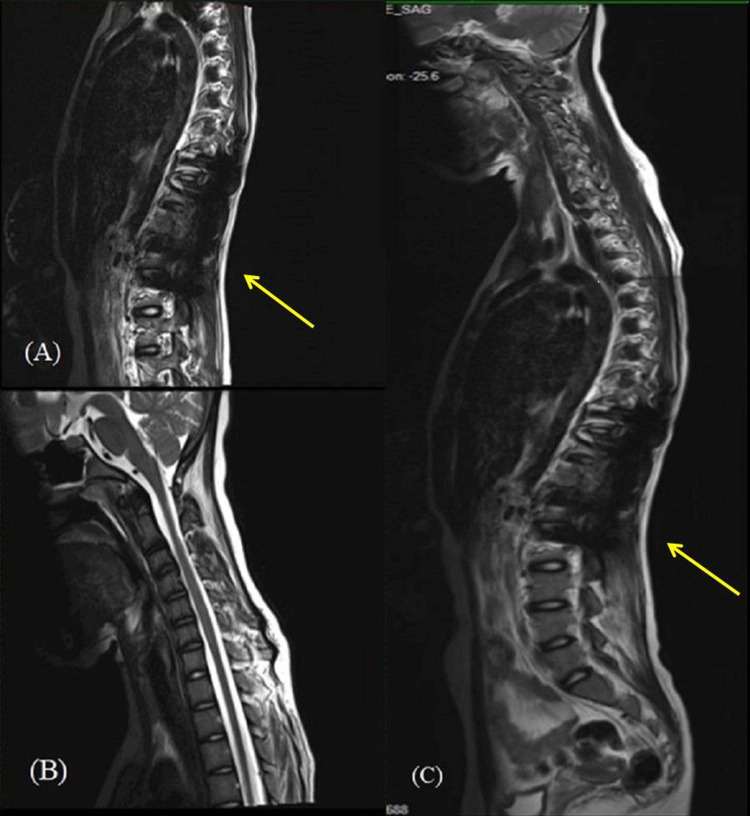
Pre-operative MRI of an aggressive lesion of the T12 vertebra (arrow); the large soft-tissue component and intraspinal extension led to spinal cord compression, resulting in cord edema.
